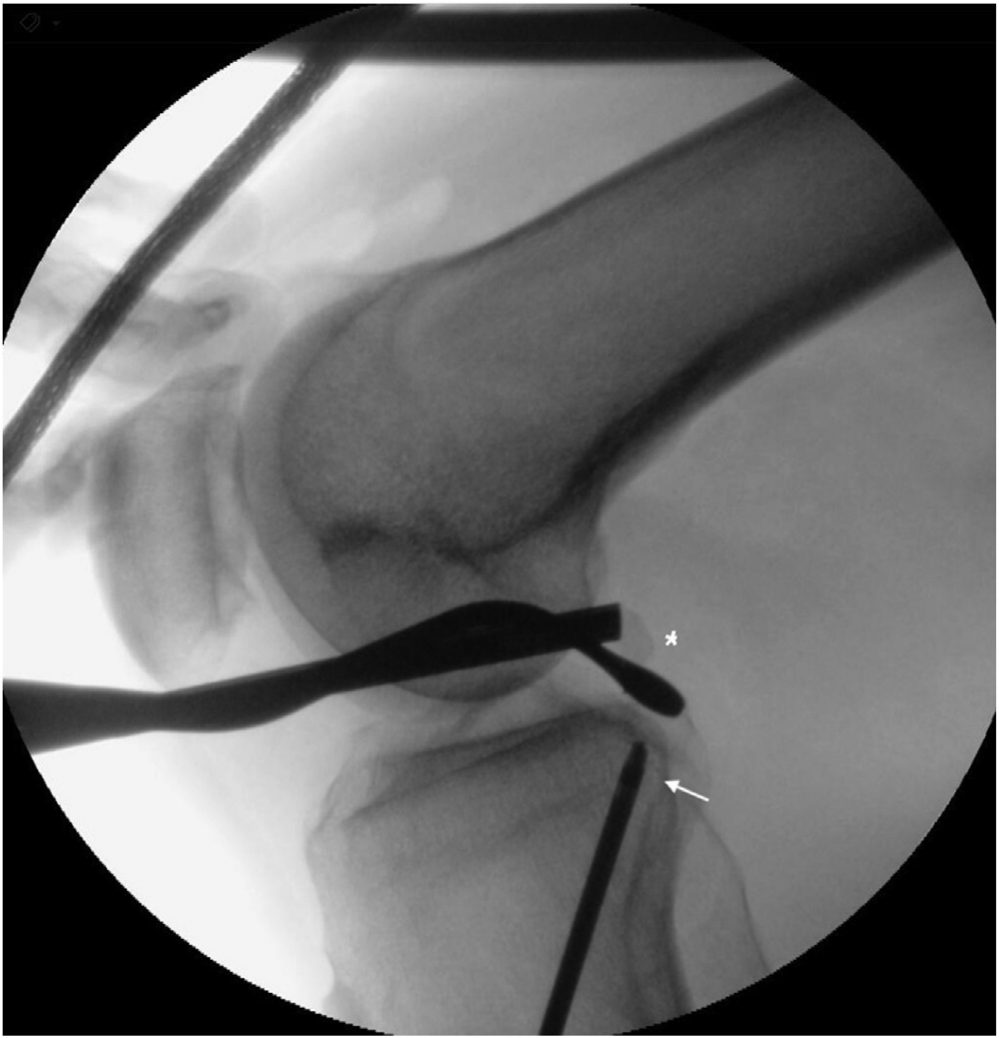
Intraoperative radiograph check for tibial PCL guide. It is highly recommended to ensure anatomical PCL insertion, and, to avoid potential vascular injuries, at this stage intraoperative radiograph should be mandatory. In the radiograph, the PCL tibial guide (white ∗) is aiming at the tibial PCL footprint, the FlipCutter (white arrow) is inserted through the tibia tunnel. (PCL, posterior cruciate ligament.)
